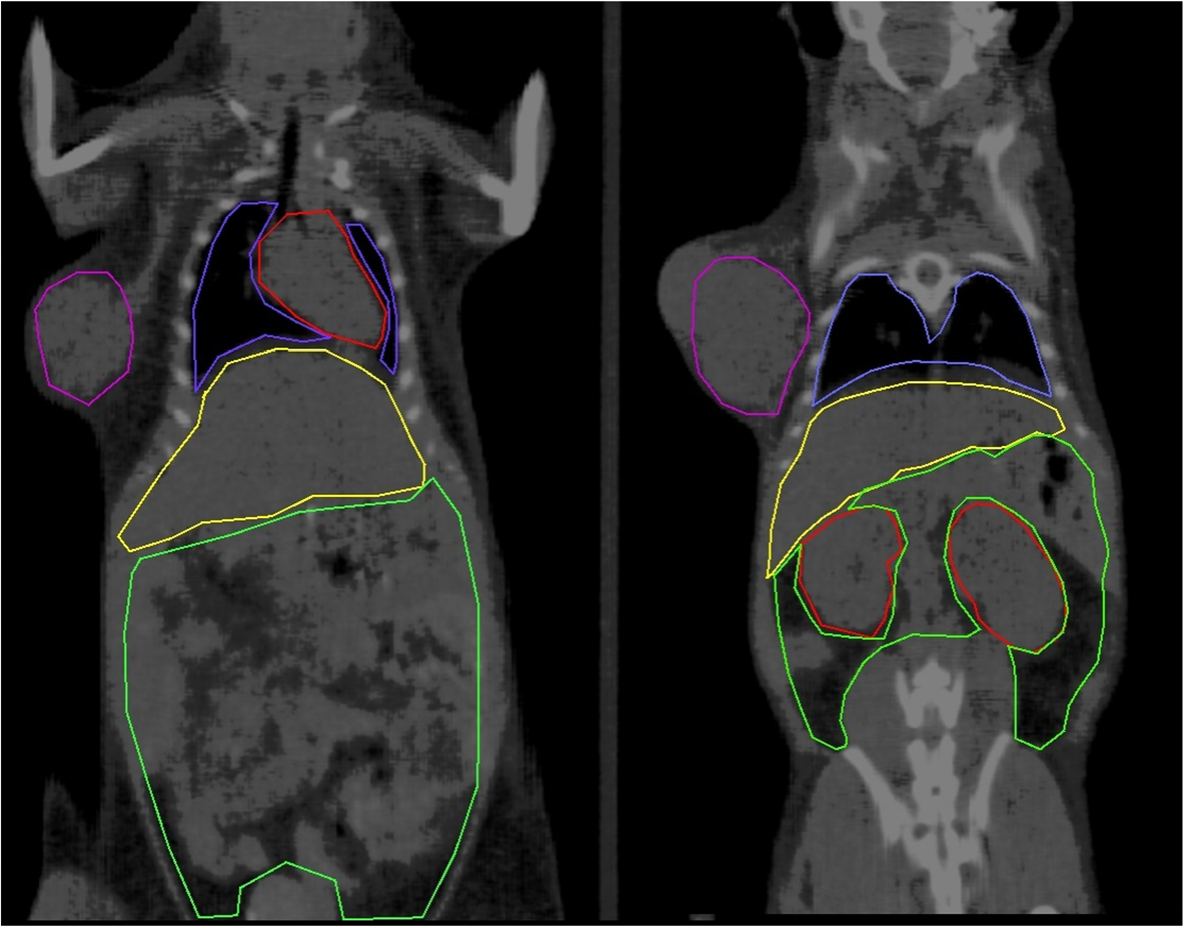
Example of manual CT-based segmentation of most relevant thoracic (heart, lungs) and abdominal organs (liver, kidneys, and intestines), along with a subcutaneous tumor on the right flank
